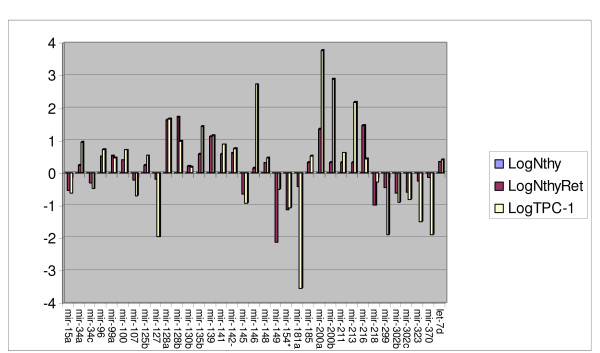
Fold Change of miRNAs in ret/PTC harbouring cell lines Vs Normal. Delta delta CT was performed using Nthy-ori 3-1 as a normal control. The log of the RQ values was used to plot the relative fold change of Nthy-Ret1 and TPC-1 against Nthy-ori 3-1. miRNAs are on the x axis.
